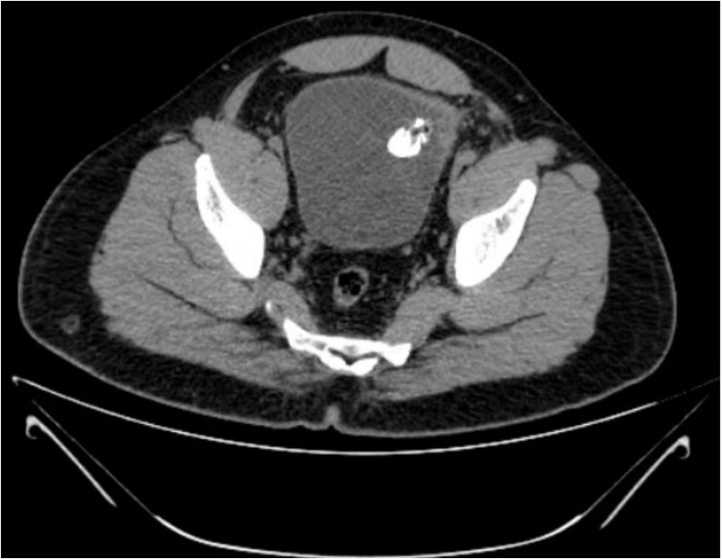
CT scan showing intraluminal calcified polypoid lesion.
